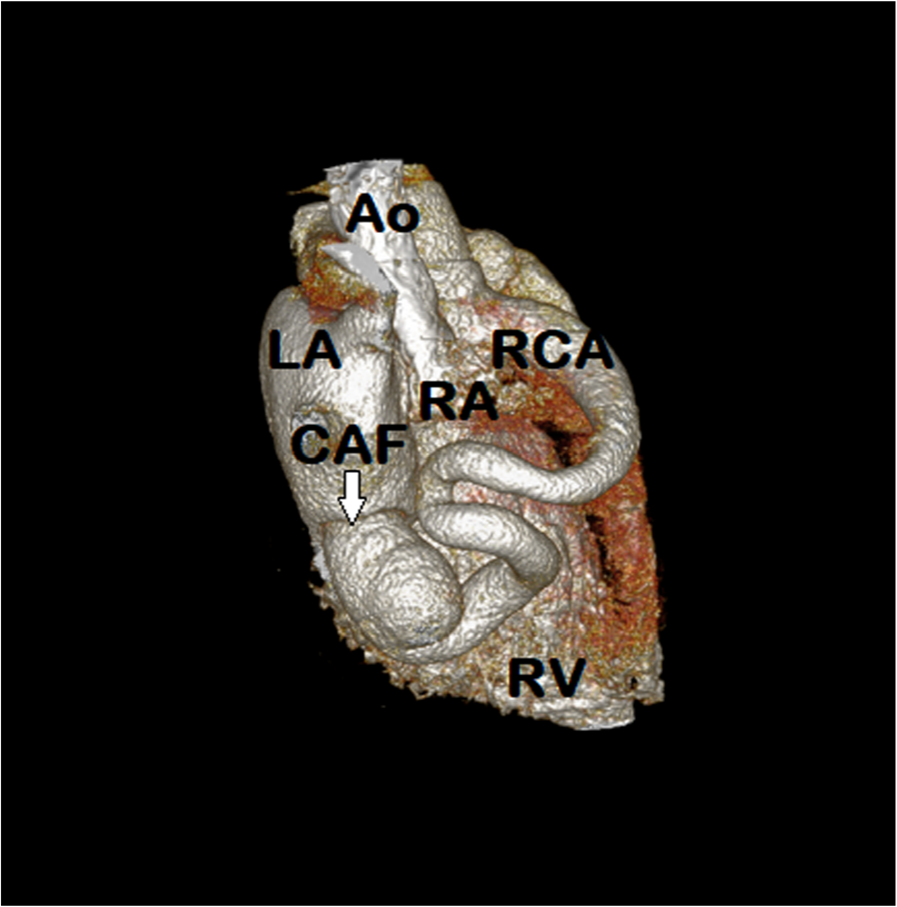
A CT (3D)-reconstruction of the heart showing dilated and tortuous right coronary artery originating from the ascending aorta, traversing through the atrioventricular groove and terminating into the floor of the left atrium. Ao- Ascending aorta, LA-Left atrium, RA- Right atrium, RCA-Right coronary artery, CAF- Coronary artery fistula, RV-Right ventricle
